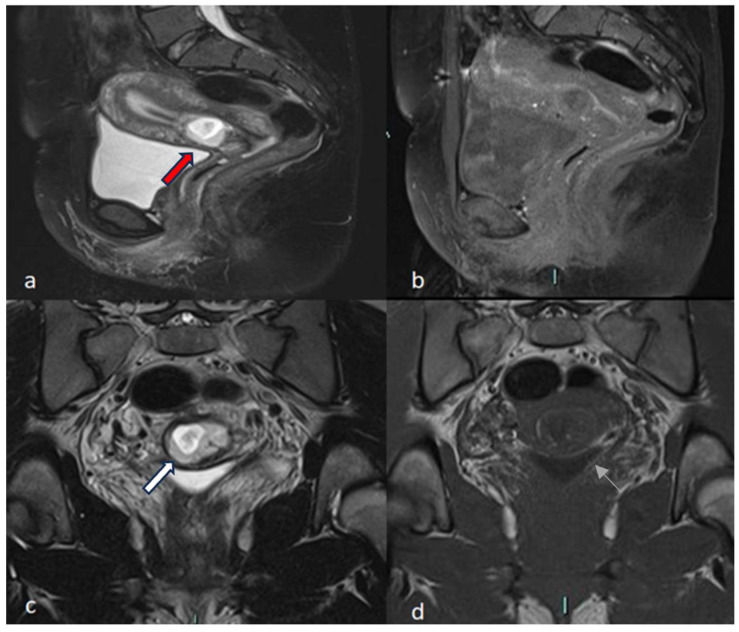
CSP Type I. Sagittal T2-weighted and fat-saturation (a,b) and axial T2-weighted and T1-weighted (c,d) MRI sequences of the pelvis showed anterior fetal pole with implant in the scar of the previous cesarean section protruding into the bladder (red arrow). A clear cleavage point with the posterior wall of the bladder was not visible. The sac was covered by a thin, hypointense layer of serosa (white arrow), and a focal interruption in the uterus serosa at anterolateral location (thin white arrow) was seen.
